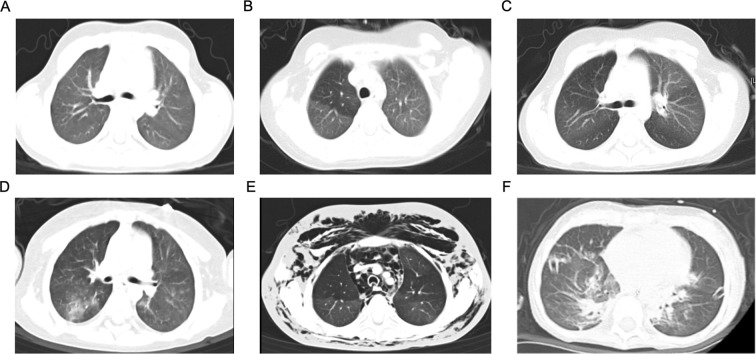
Chest CT findings of HSCT. (A) Axial chest CT prior to HSCT showing unremarkable lung parenchyma. (B, C) Axial chest CT during BOS episode post-HSCT demonstrating mosaic perfusion pattern (B) and bronchial wall thickening (C, D) Axial chest CT during a post-HSCT pulmonary infection episode revealing corresponding infectious infiltrates. (E) Axial chest CT after development of ALS, showing bilateral atelectasis, extensive subcutaneous emphysema, pneumomediastinum, and pneumothorax. (F) Axial chest CT following lung transplantation. HSCT, hematopoietic stem cell transplantation; BOS, bronchiolitis obliterans syndrome; ALS, air-leak syndrome.
